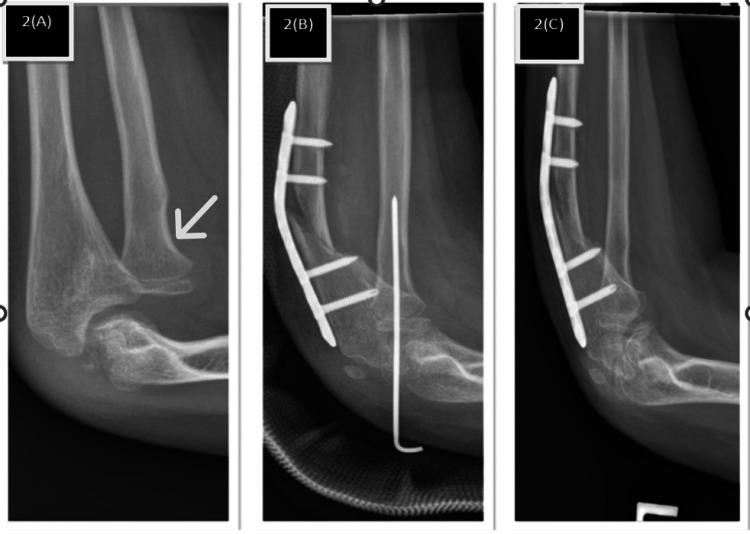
Plain radiograph of patient 2. (2A) Bado type 1 with no obvious deformity of the ulna. (2B) Post-ulna sliding osteotomy with transcapitellar K-wire fixation of the radial head. (2C) The radial head remained reduced after K-wire removal with united ulnar osteotomy.
